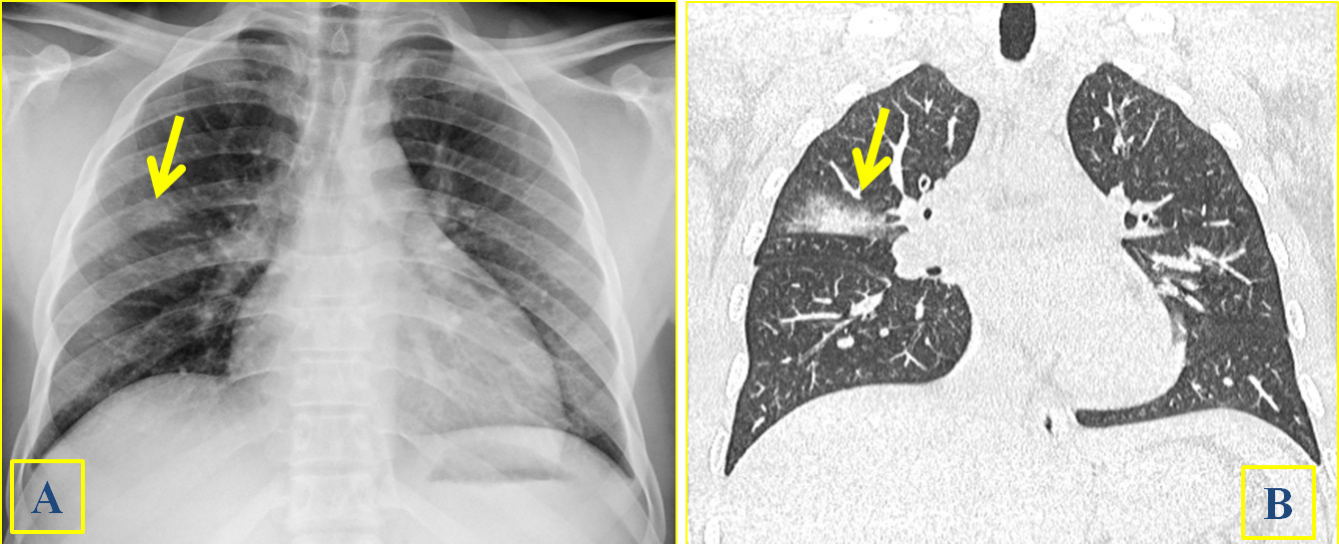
A 28-year-old male with clinical suspect of schistosomiasis (3A-3B). Lung consolidation (arrows) can be identified close to fissure at CR (3A) and at HRCT (3B).
